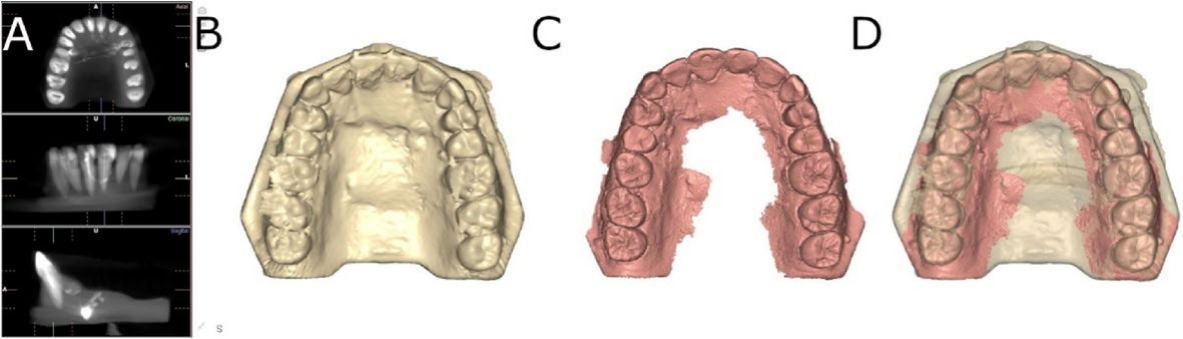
A DICOM files from the CBCT scan, B render STL digital file from the CBCT scan, C STL digital file from the digital impression and D alignment procedure between STL and CBCT scan digital files
